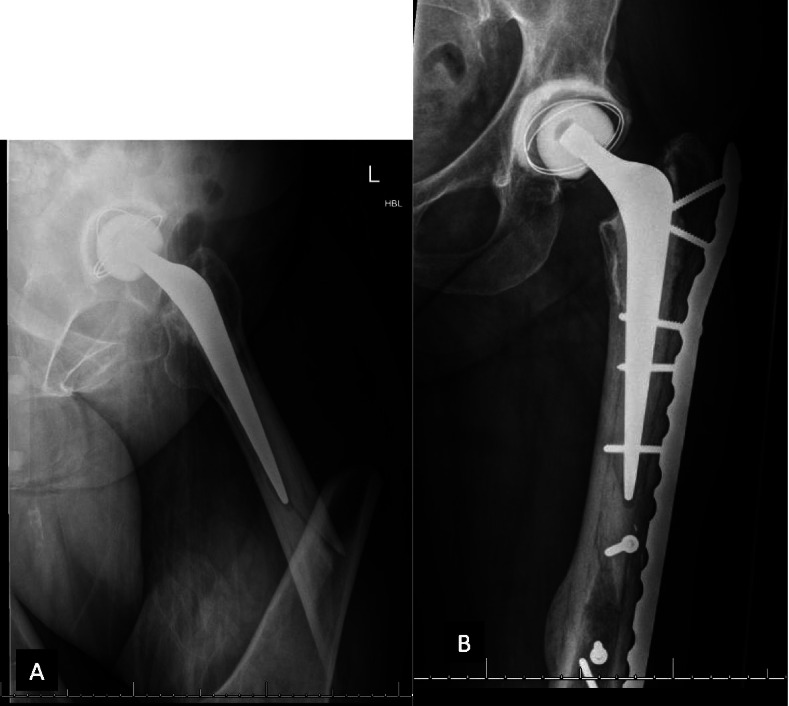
A periprosthetic femoral fracture. A Preoperative X-ray. B The fracture is fixed with a locking plate
